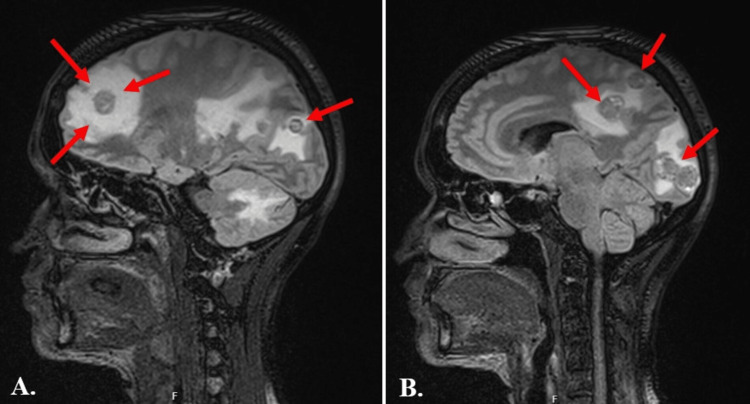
Contrast-enhanced magnetic resonance imaging of the brain (sagittal sections) showing multiple heterogeneously enhancing metastatic lesions with surrounding vasogenic oedema. (A) Lesions involving the frontal and occipital lobes (arrows). (B) Lesions involving the temporal, parietal, and occipital lobes with associated mass effect (arrows).
